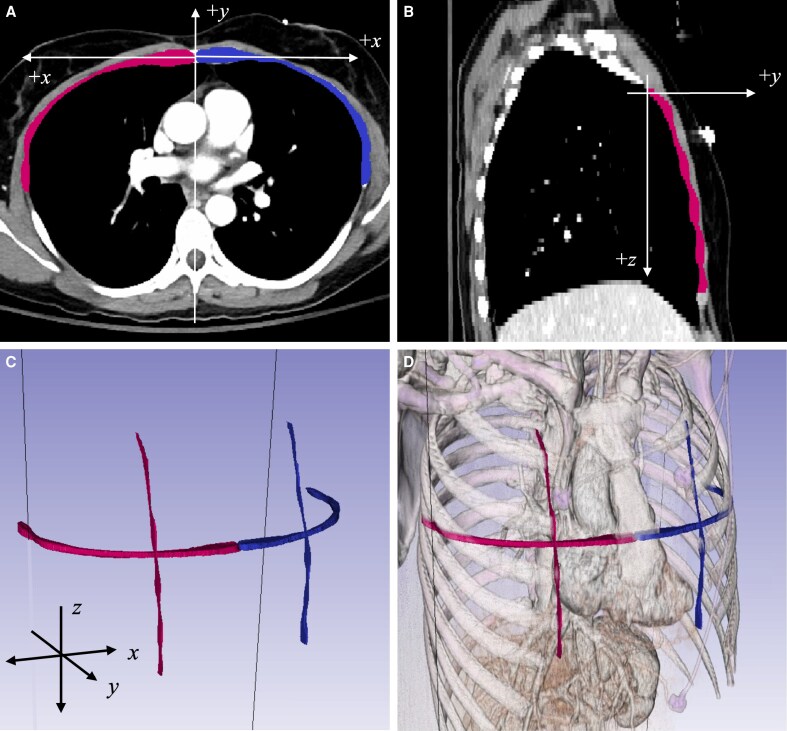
Segmentation of the bony thorax. (A) Axial CT slice showing the horizontal segmentation plane at the level of the fourth rib insertion into the sternum. The thorax is divided into right and left segments. (B) Sagittal CT slice demonstrating the vertical segmentation plane, placed at one-quarter of the thorax width at the same level. (C) Three-dimensional representation of the segmentation planes with orientation shown in *x*, *y*, and *z* axes. (D) Anatomical 3D rendering of the segmentation planes overlaid on thoracic anatomy, excluding chest wall musculature.
